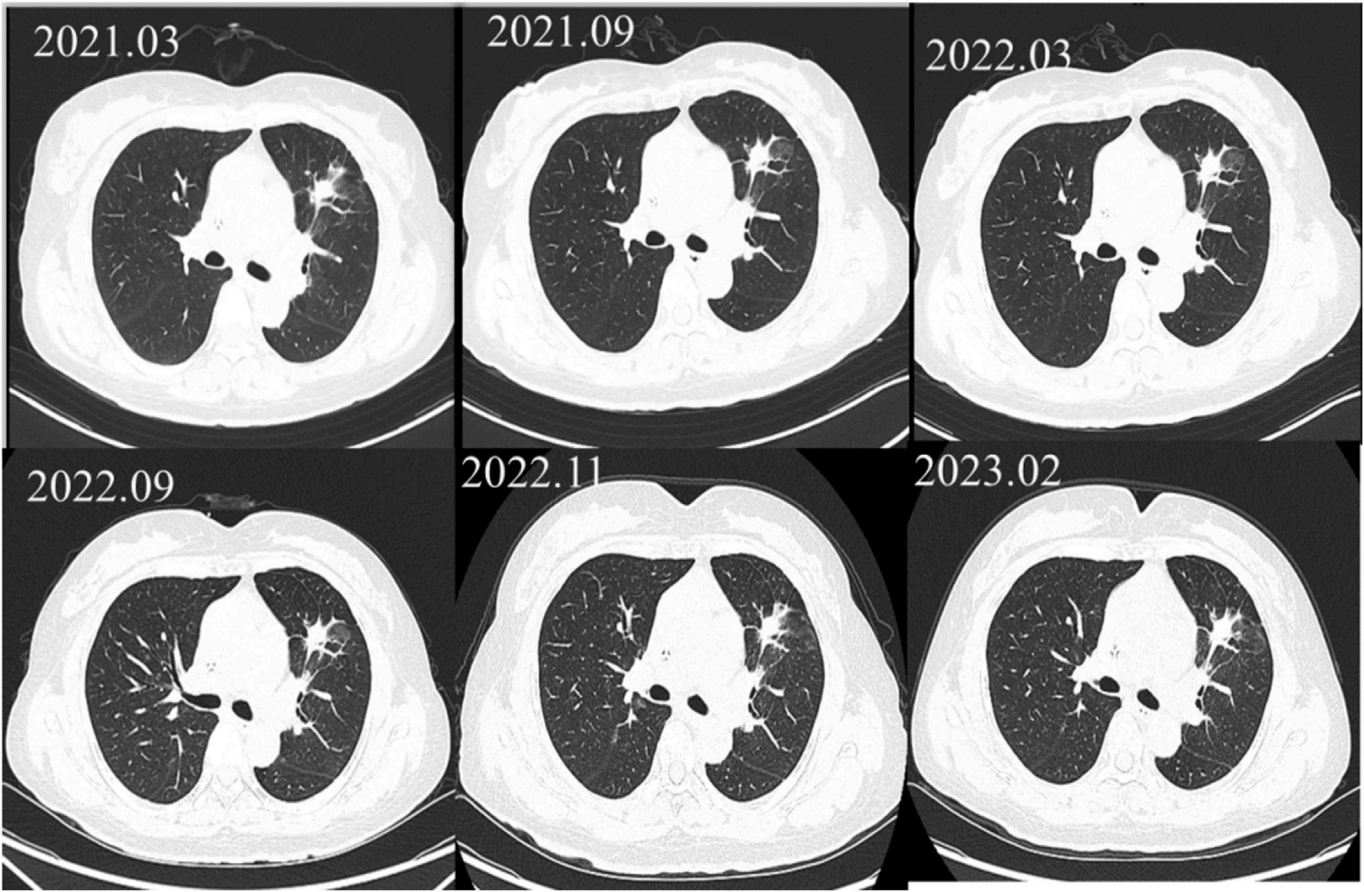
The patient’s chest computed tomography (CT) scans.
